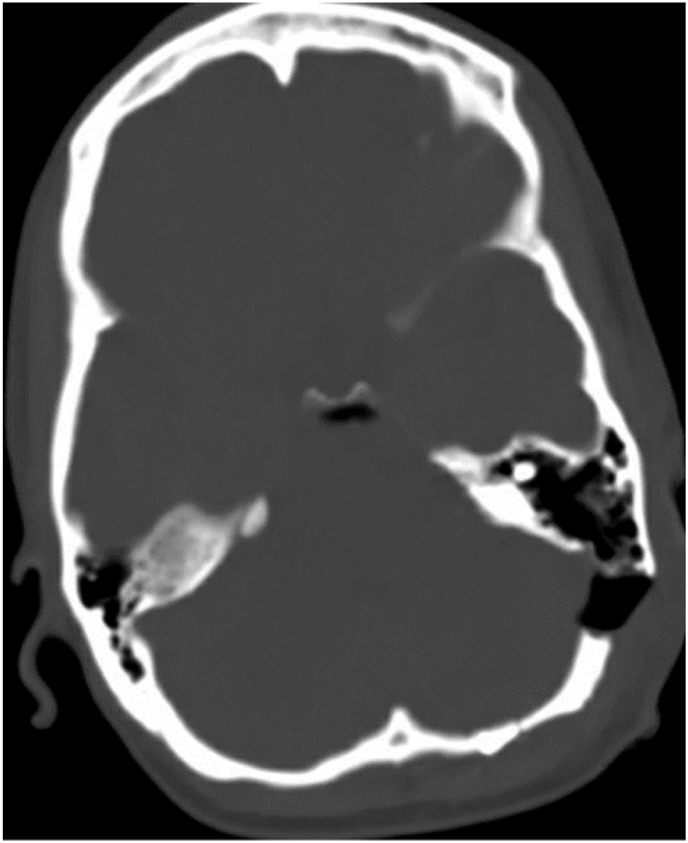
Post-operative CT-scan bone window, showing complete drilling of left suprameatal tubercle.
